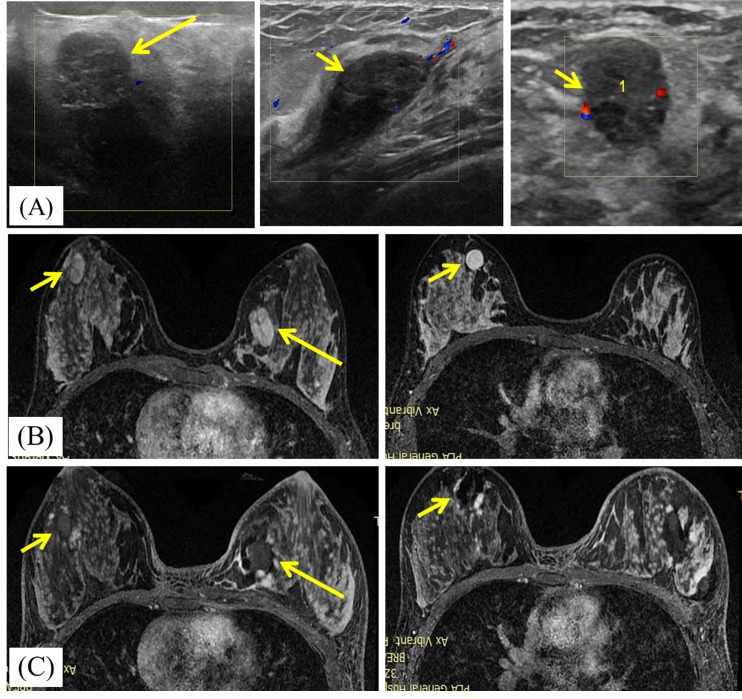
A 23-year-old woman with bilateral breast fibroadenomas A. Ultrasound scan before microwave ablation(MWA) shows the three hypoechoic BBLs with poor blood flow signal. The masses size are 2.8cmx1.3cmx1.5cm (in left breast, large arrows), 1.5cmx1.4cmx1.2cm (in right breast, small arrows) and 2.2cmx1.0cmx2.1cm (in right breast, small arrows), respectively. B. Transverse contrast-enhanced magnetic resonance imaging (MRI) shows hyperintensity masses in left breast (large arrows) and right breast (small arrows) before MWA in arterial phase. C. Contrast-enhanced MRI image shows hypointensity MWA treatment zone (arrows) for bilateral breast fibroadenomas in arterial phase.
